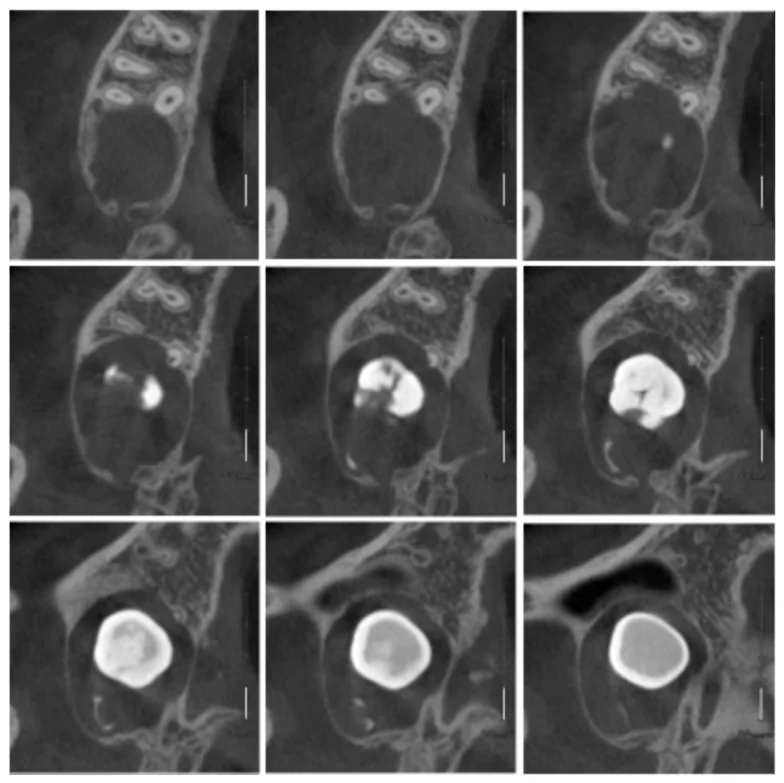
Axial section of CBCT images at 12 years 6 months of age. Scale bar = 5 mm. This revealed the buccal distal root and palatal root were connected to the lesion and exhibited resorption. In addition, cortical bone was thinned and partial disruption was observed.
